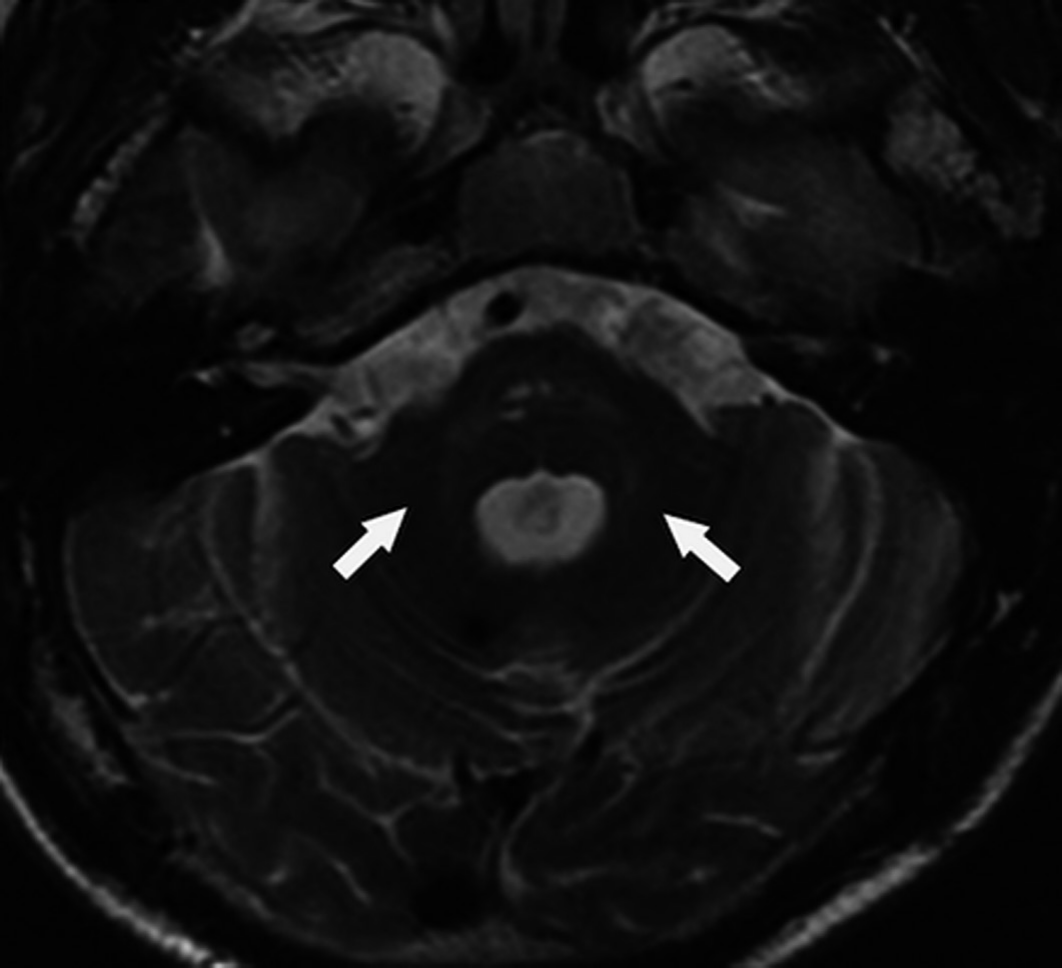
Example of the “arc sign” of the cerebellopontine peduncle on MRI imaging. Reproduced with permission from Yu et al [^22^]. Copyright © 2020 Elsevier.
